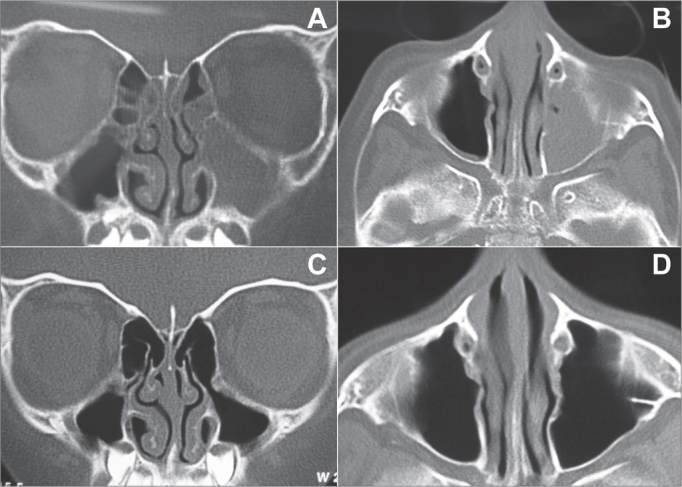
Pre and post-operative CT scan (exam carried out 6 months after the surgery) from a patient submitted to sinuplasty. A: Coronal section of a pre-operative image showing complete blurring of the left maxillary sinus and some ipsilateral ethmoidal cells. B: axial section of a pre-operative image showing blurring of the left maxillary sinus. C: post-operative image (6 months after the procedure) in a coronal cross-section showing normal aeration of the left maxillary sinus and ipsilateral ethmoidal cells. Notice the dilatation seen in the region of the maxillary infundibulum. D: Post-operative image (6 months after the procedure) in an axial cross-section showing normal aeration of the left maxillary sinus.
